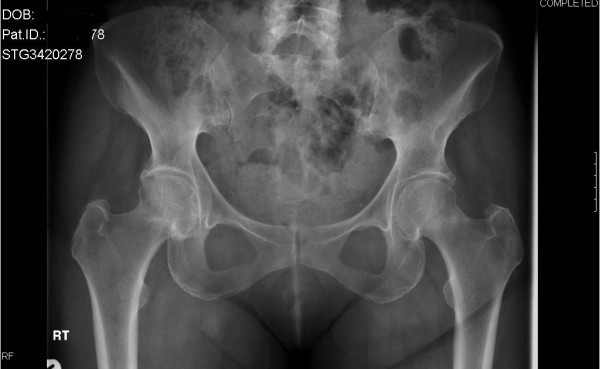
Three month follow-up plain radiograph showing healed undisplaced neck of femur fractures.
